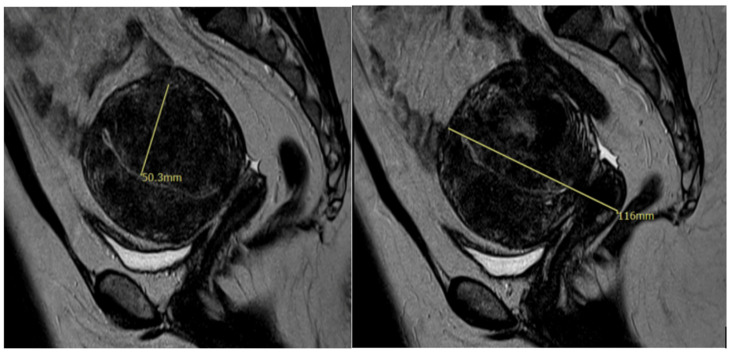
MRI image showing diffuse adenomyosis.
